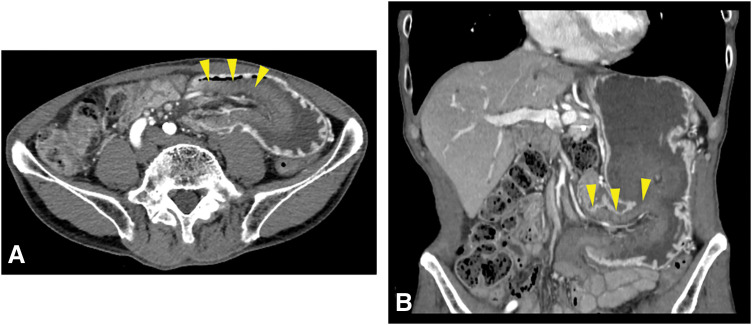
Contrast-enhanced abdominal CT in both an axial image (A) and a coronal image (B) revealed jejunal loop intussusception at the gastrojejunal anastomosis (yellow arrowheads), which had evidence of edematous wall with slightly poor enhancement of the intussuscepted loop.
